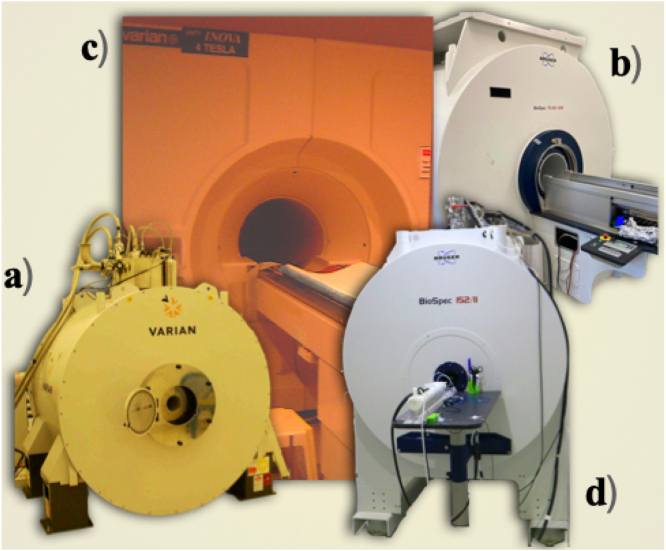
MRI systems used to experimentally test the in-house and vendor-provided birdcage (BC) coils at different resonant frequencies: (a) a 7 T/12 cm imager from Agilent (Varian, Santa Clara, CA) with actively shielded gradients (400 mT/m maximum strength); (b) a 7 T/30 cm imager from Bruker BioSpin (GmbH, Ettlingen, Germany) with high-performance gradients (1000 mT/m strength, 9000 T/m/s slew rate); (c) a 4 T/60 cm imager using an INOVA (Varian, Santa Clara, CA) console with Siemens SONATA gradients (70 mT/m strength, 200 T/m/s slew rate); and (d) a 15.2 T/11 cm imager from Bruker BioSpin with an actively shielded gradient system (1000 mT/m strength, 9000 T/m/s slew rate).
